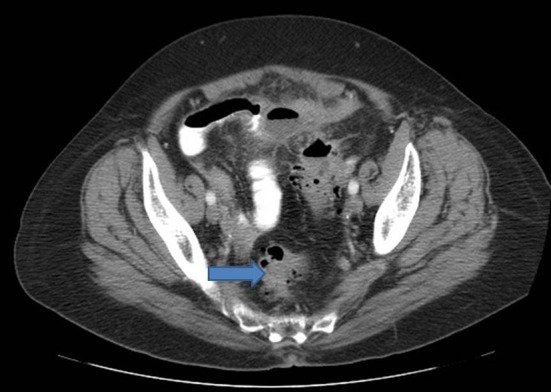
CT scan of abdomen showing thickening of the rectal mucosa.
